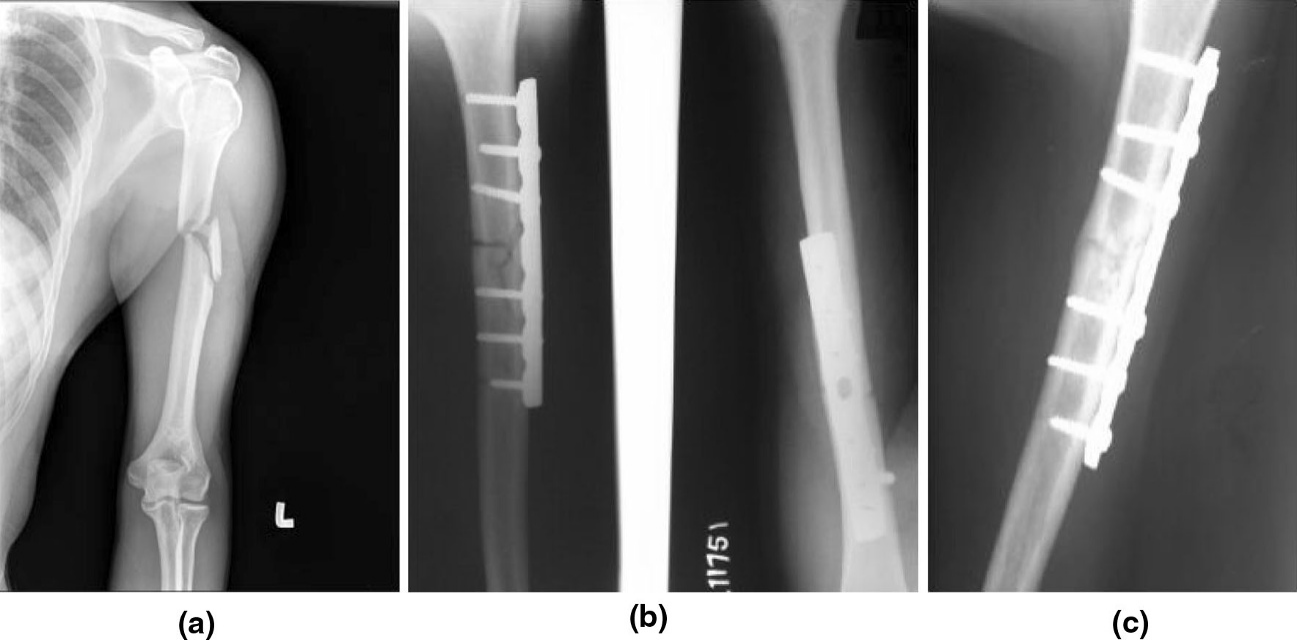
a Preoperative X-ray of 30-year-old male patient showing displaced fracture of the shaft of the humerus, left side. b Immediate postoperative X-ray anteroposterior (AP) and lateral view showing plate osteosynthesis with 4.5-mm narrow LCDCP. c Postoperative X-ray at 12-week follow-up visit showing a well-uniting fracture
